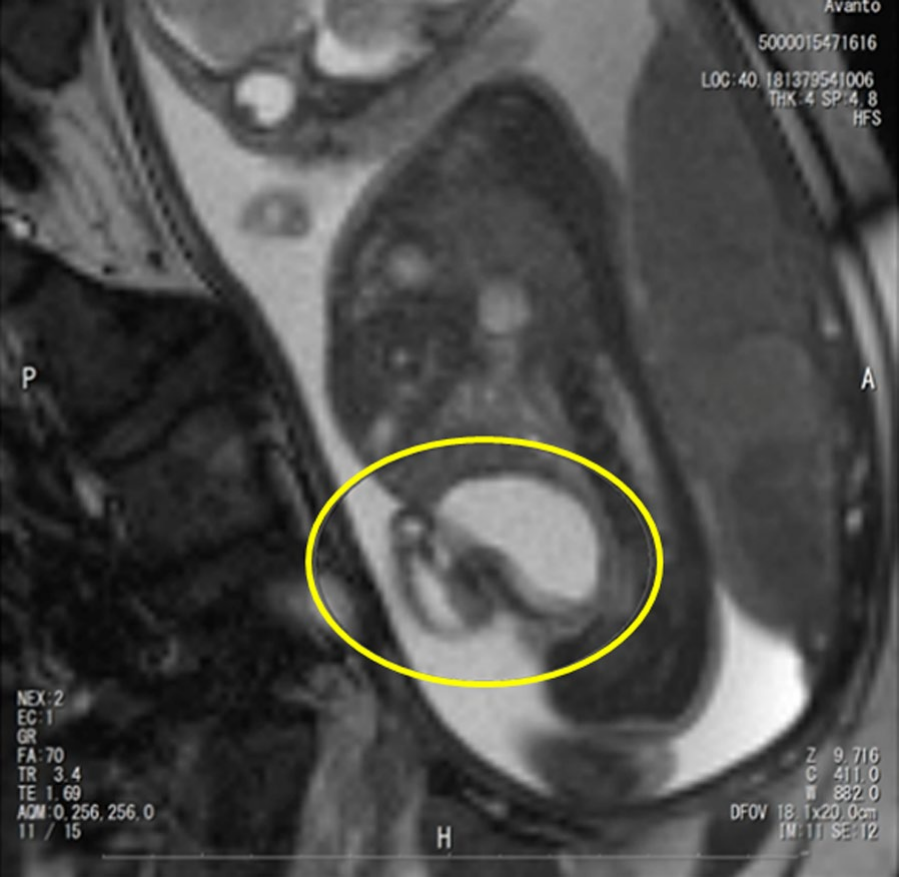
Fetal MRI finding of umbilical cyst at 25 weeks gestation. Yellow circle shows fetal bladder and umbilical cyst
